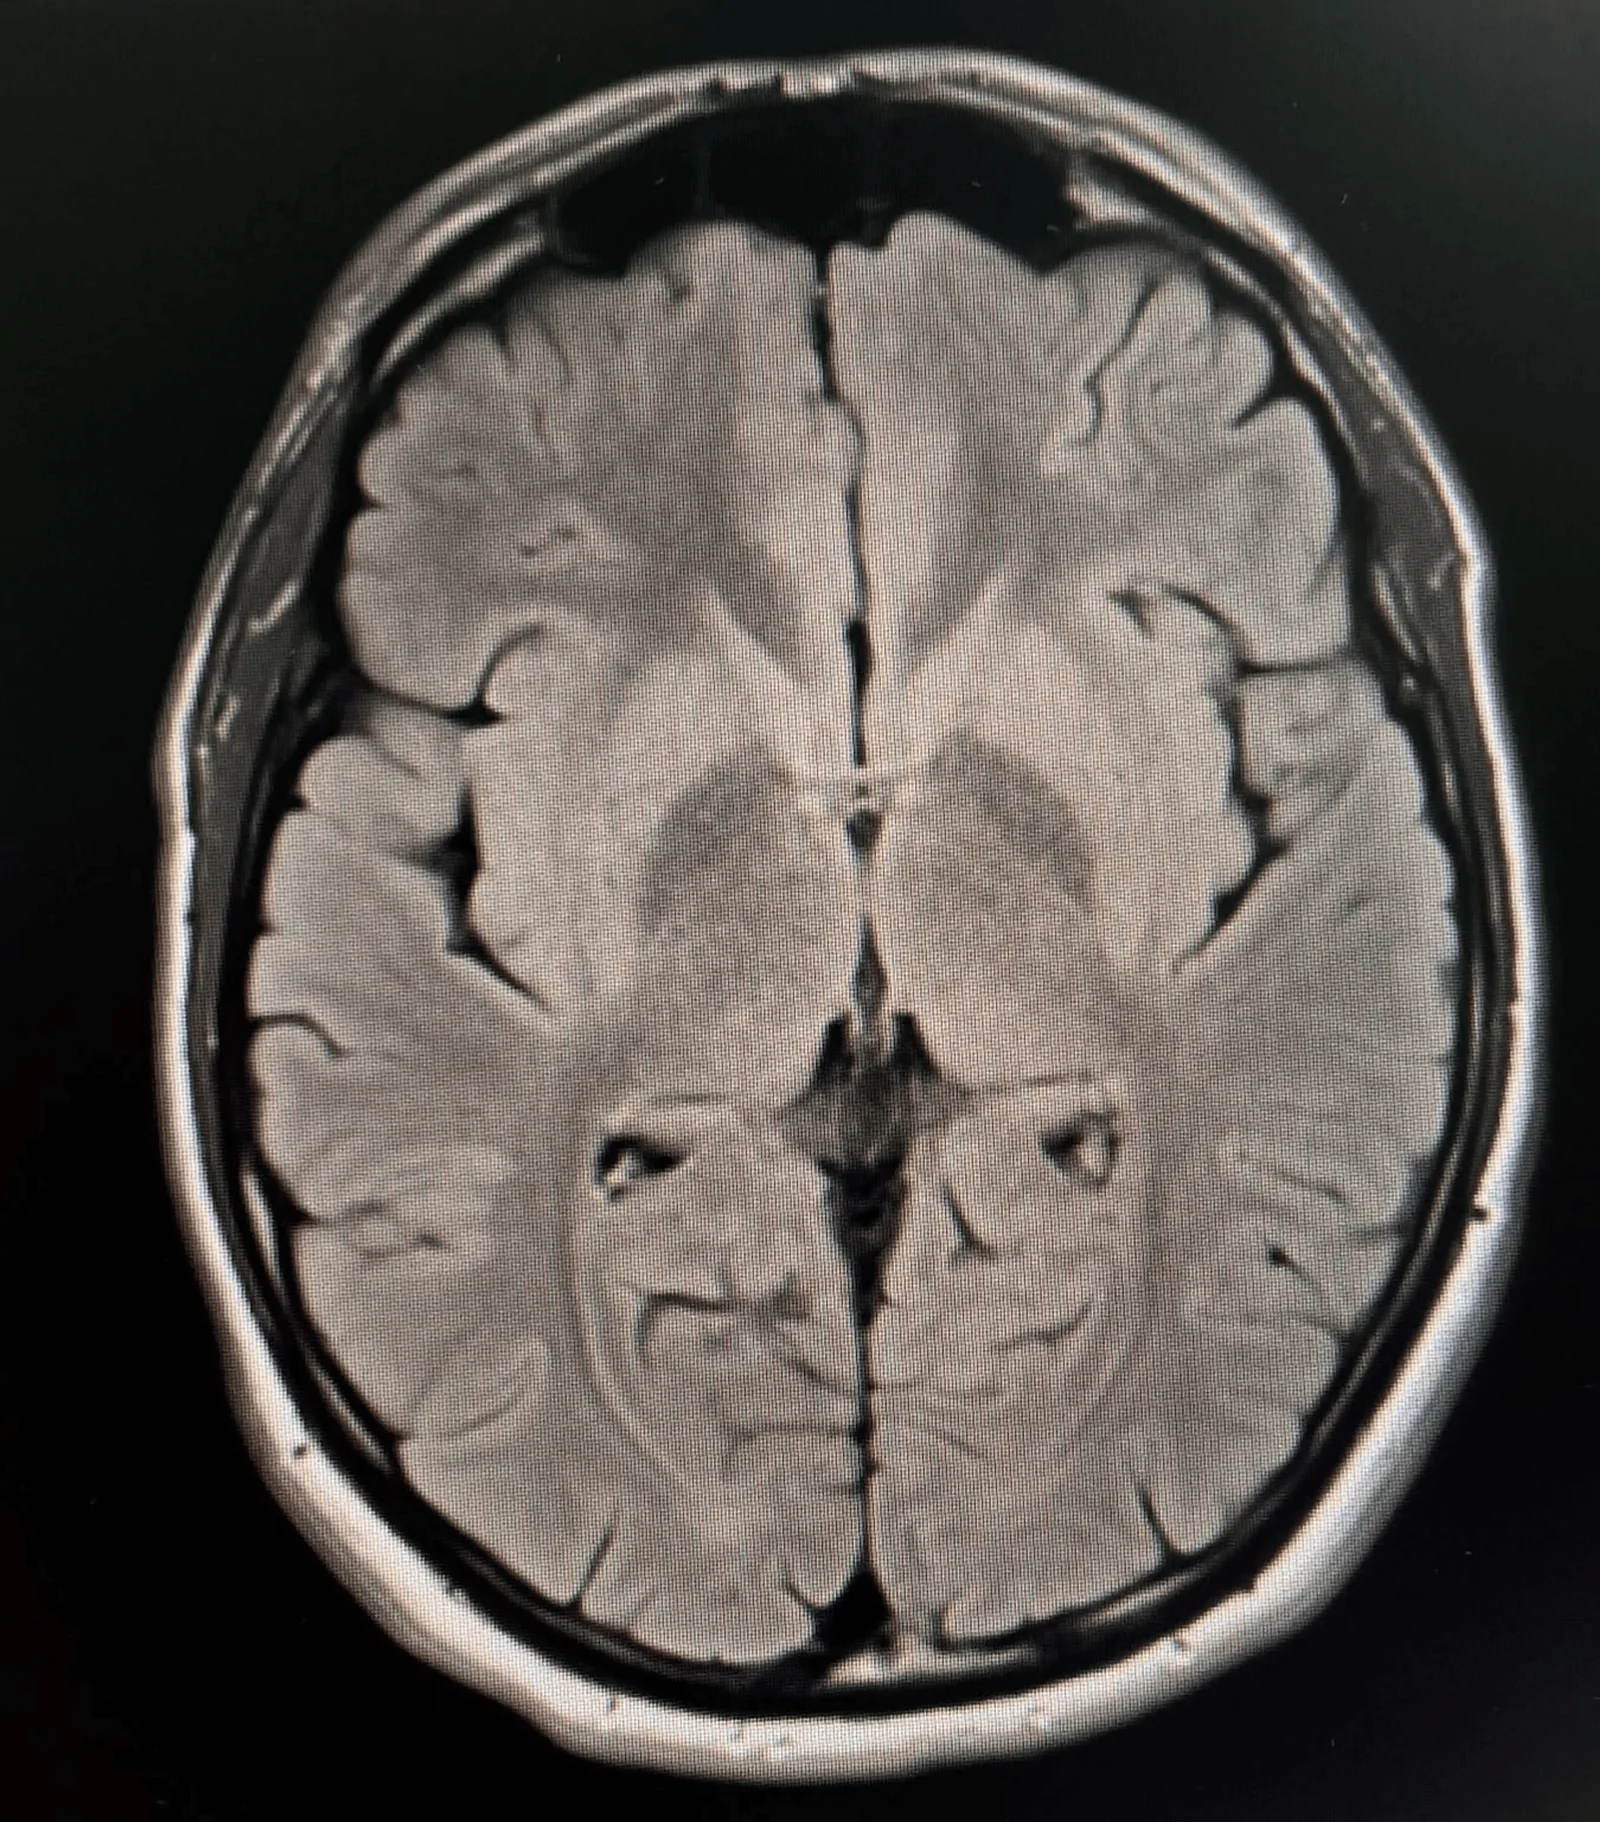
Magnetic resonance imaging demonstrating a normal study Magnetic resonance imaging of the brain demonstrating no evidence of cerebral infarction, intracranial hemorrhage, hydrocephalus, or cerebral abscess
